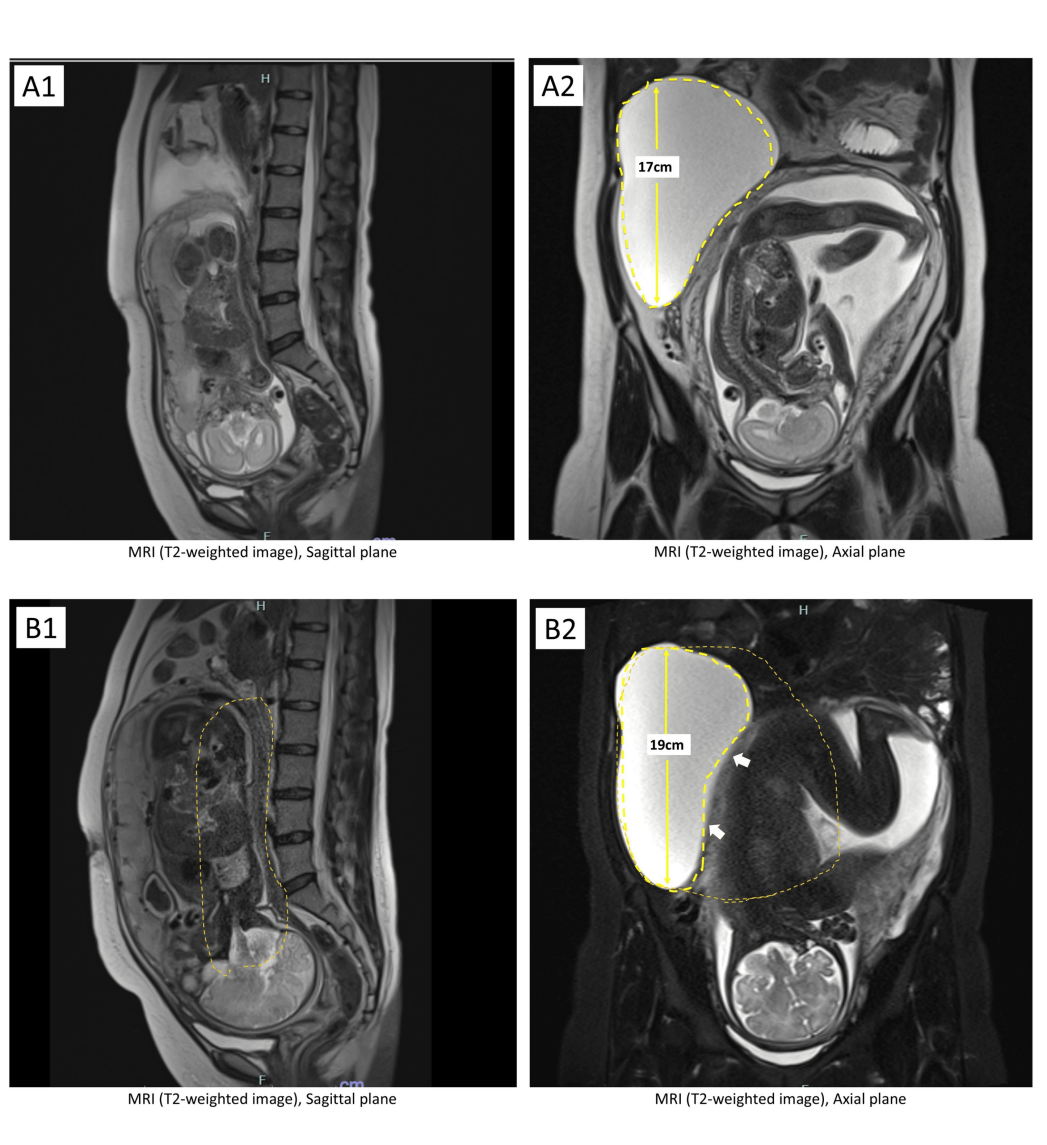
MRI at 25 and 34 weeks’ gestation A1.2:  MRI at 25 weeks’ gestation. The cyst is flexibly displaced to the right upper area of the uterus without significant changes in size. B1.2:  MRI at 34 weeks’ gestation. There is no abdominal distension or respiratory distress, and the cyst size remains unchanged.
